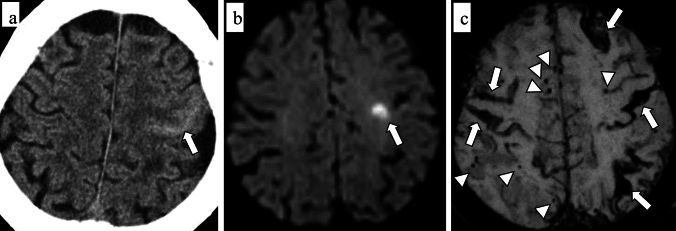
An 84 year-old female with probable CAA. She complained of recurrent episodes of numbness in her right upper extremity, which were considered to represent TFNE. CT (a) detects convexity subarachnoid hemorrhage (cSAH) in the central sulcus (arrow). Diffusion-weighted image (DWI) (b) shows a small DWI lesion (arrow) in the left frontal lobe. On SWI (c), disseminated cSS (arrows) and multiple CMBs (arrowheads) in bilateral hemispheres
